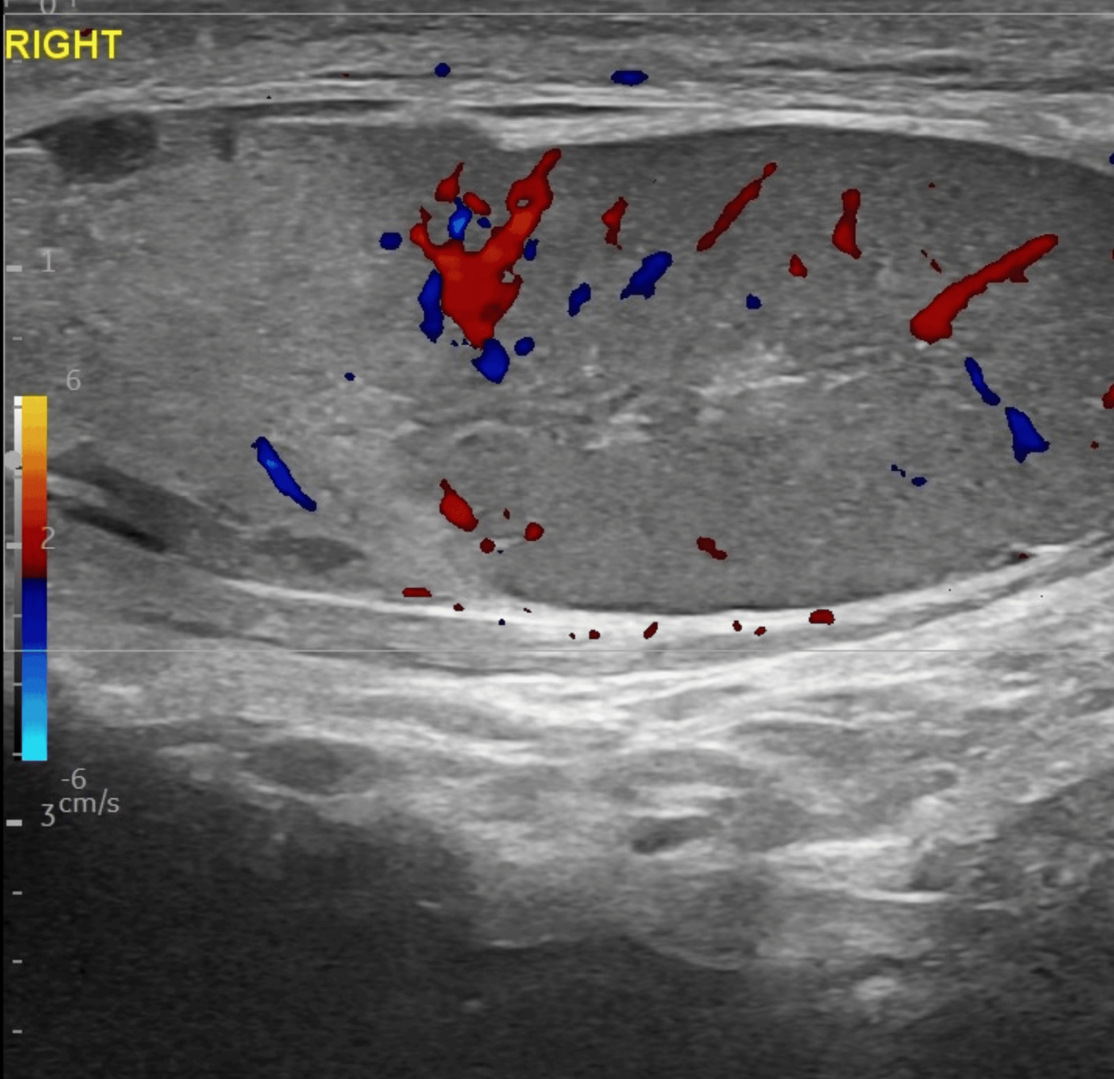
Testicular heterogeneity. The sagittal ultrasound color Doppler plane demonstrated a heterogeneous and avascular echotexture of the upper pole of the right testis in the setting of a larger testicular hematoma contusion.
